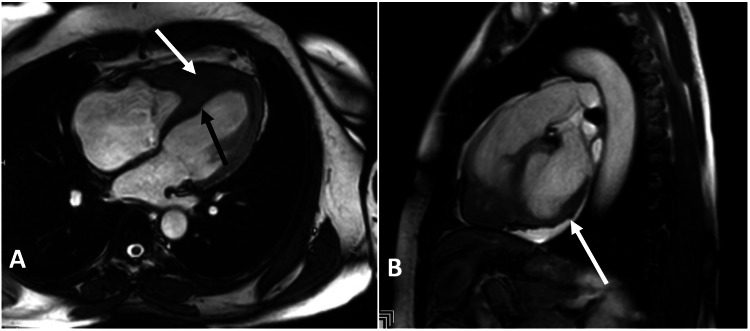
Cardiac MRI, CINE 4 chamber clip (A), and CINE RVOT clip (B) show mid-to-apical LV septal hypertrophy (black arrow) along with RV wall thickening (white arrows). LV: left ventricle, RV: right ventricle
